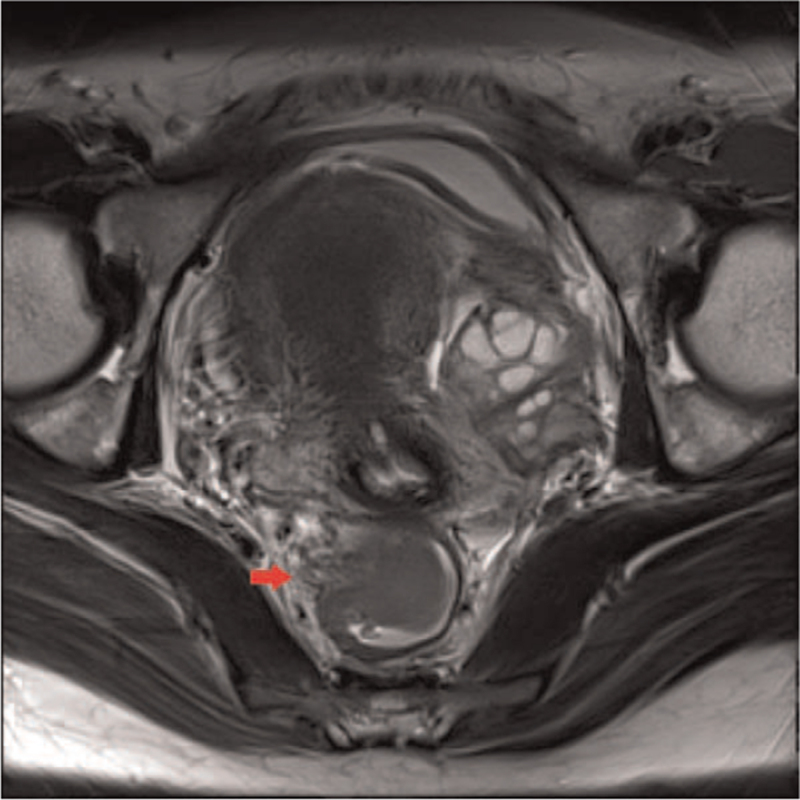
Rectal magnetic resonance imaging shows a 4.0 × 3.0-cm-sized well-defined intraluminal mass with intact overlying mucosa in the right wall of the rectum with suspicious right perirectal fat infiltration (red arrow).
